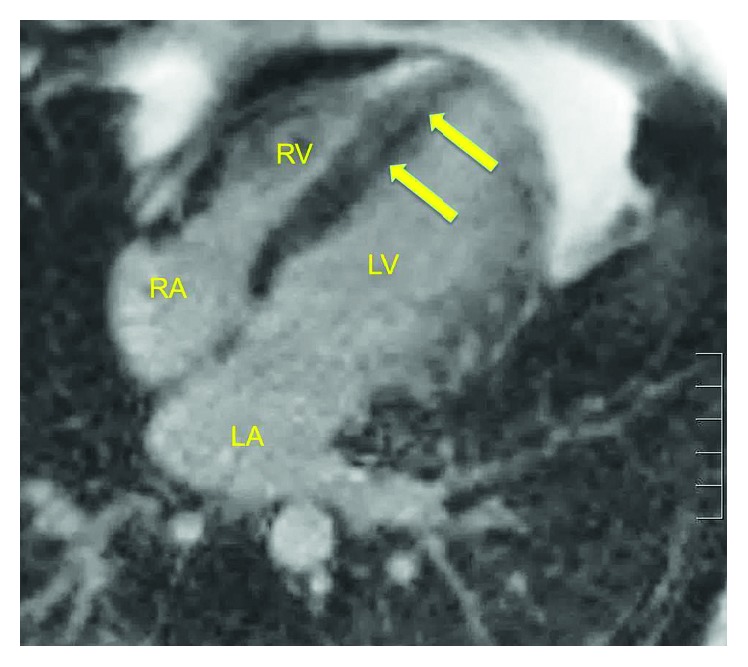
Magnitude reconstructed inversion recovery image of MRI T1-weighted imaging depicting linear delayed hyper-enhancement involving the midmyocardium of the distal septum. This translates to gadolinium lighting up in the midmyocardium due to loss of cellular integrity.
